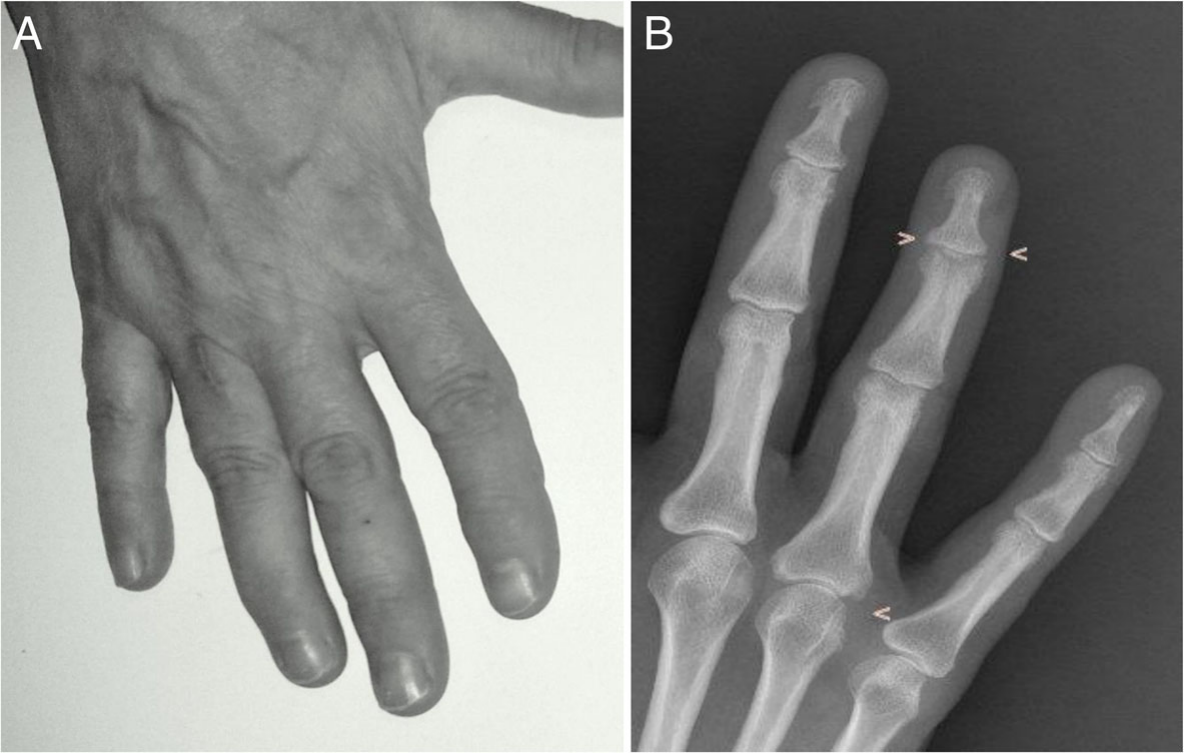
Right hand:swelling of the fourth distal interphalangeal joint was markedly improved, and nail appeared normal (a). Radiograph of the right hand: the bone erosion in the fourth distal interphalangeal joint seemed to have disappeared (b)
